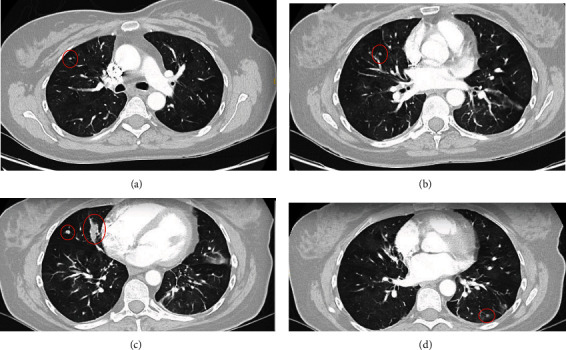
CT chest with diffuse mosaic attenuation and multiple pulmonary nodules: (a) 4 mm right upper lobe nodule; (b) 4 mm right middle lobe nodule; (c) 7 mm lateral right middle lobe nodule with confluent 1.8 cm medial right middle lobe nodule; (d) 4 mm left lower lobe nodule.
